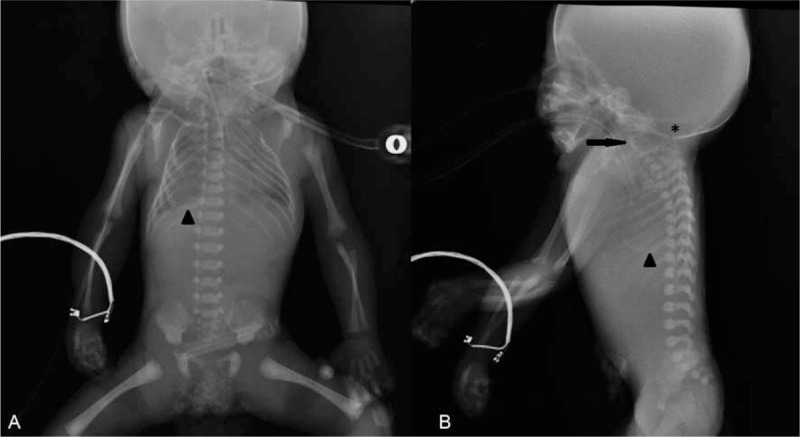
Frontal (A) radiograph of case 12 shows asymmetrical thoracic cage, and thin and deformed ribs. Sprengel deformity (congenital elevation of the scapula) is bilaterally present. Note that there is no passage of gas into the stomach and intestines. The orogastric tube does not pass through esophagus into the stomach, and is twisted in the thoracic cage (arrowhead). Lateral spine radiograph (B) shows fusion of adjacent cervical vertebral bodies (arrow), moderate dorsiflexion of cervical spine, and wide foramen magnum (asterisk). The calvarium is relatively large.
